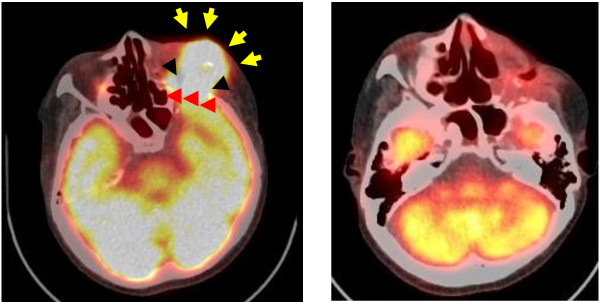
^18^FDG-PET study prior and 6 months after BNCT of a 56 year-old male patient with recurrent squamous cell carcinoma of the maxilla.A: FDG accumulated in the left orbital region (arrows) and frontal lobe of brain (arrow heads). B: No accumulation of FDG-PET was detected 6 months after BNCT and the patient was disease free for 61 months at the time of the original report. Photographs are from *Applied Radiation and Isotopes*, 67:S37-S42, 2009.
